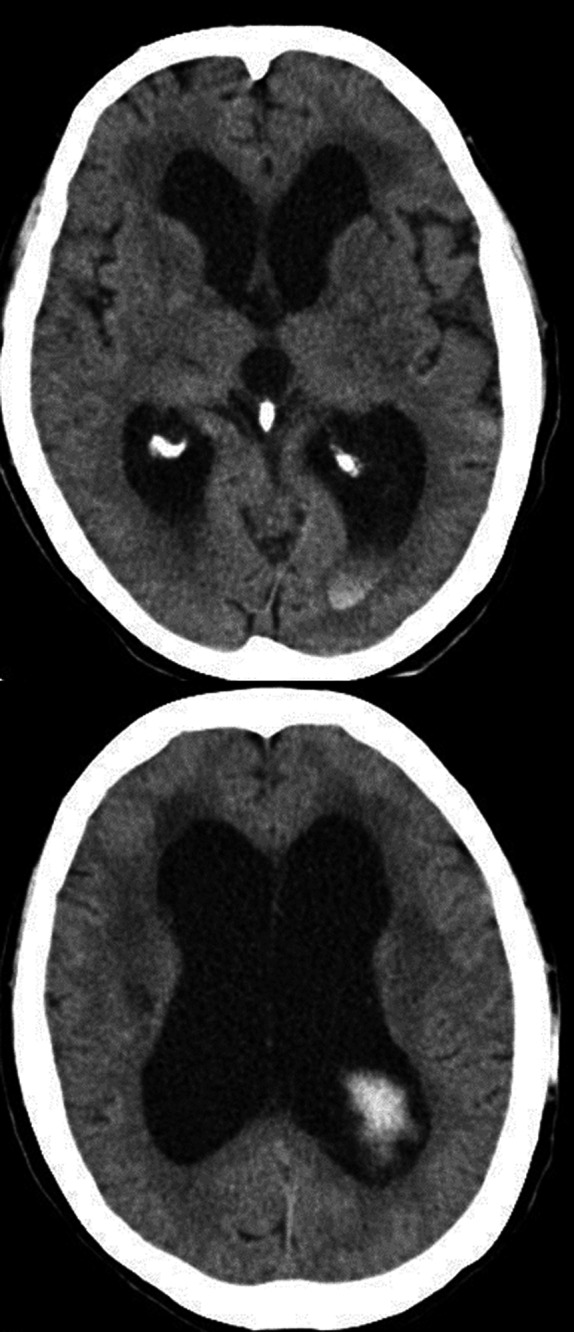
Computed tomography scan on admission showing ventricular enlargement and intraventricular hemorrhage.
